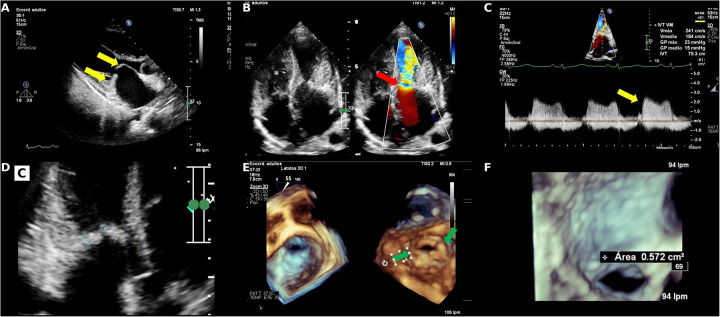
Diagnostic multimodality echocardiography at presentation. (A) Parasternal long-axis view showing diffuse mitral leaflet thickening with a hockey-stick deformity of the anterior leaflet (yellow arrows). (B) Apical four-chamber color Doppler showing a turbulent, high-velocity, mosaic diastolic jet through the narrowed orifice (red arrow), with severe biatrial enlargement. (C) Transmitral continuous-wave Doppler (yellow arrow) showing a peak velocity of 241 cm/s and a mean gradient of 15 mmHg. (D) Two-dimensional planimetry of the mitral orifice (0.84 by 0.80 cm), with diffuse leaflet thickening and commissural fusion. (E) Three-dimensional transesophageal echocardiography (3D TEE), en-face view from the left atrial side, showing the fish-mouth commissural fusion of the stenotic orifice (green arrows). (F) 3D TEE direct planimetry of the mitral orifice, giving an effective orifice area of 0.572 cm^2^.
